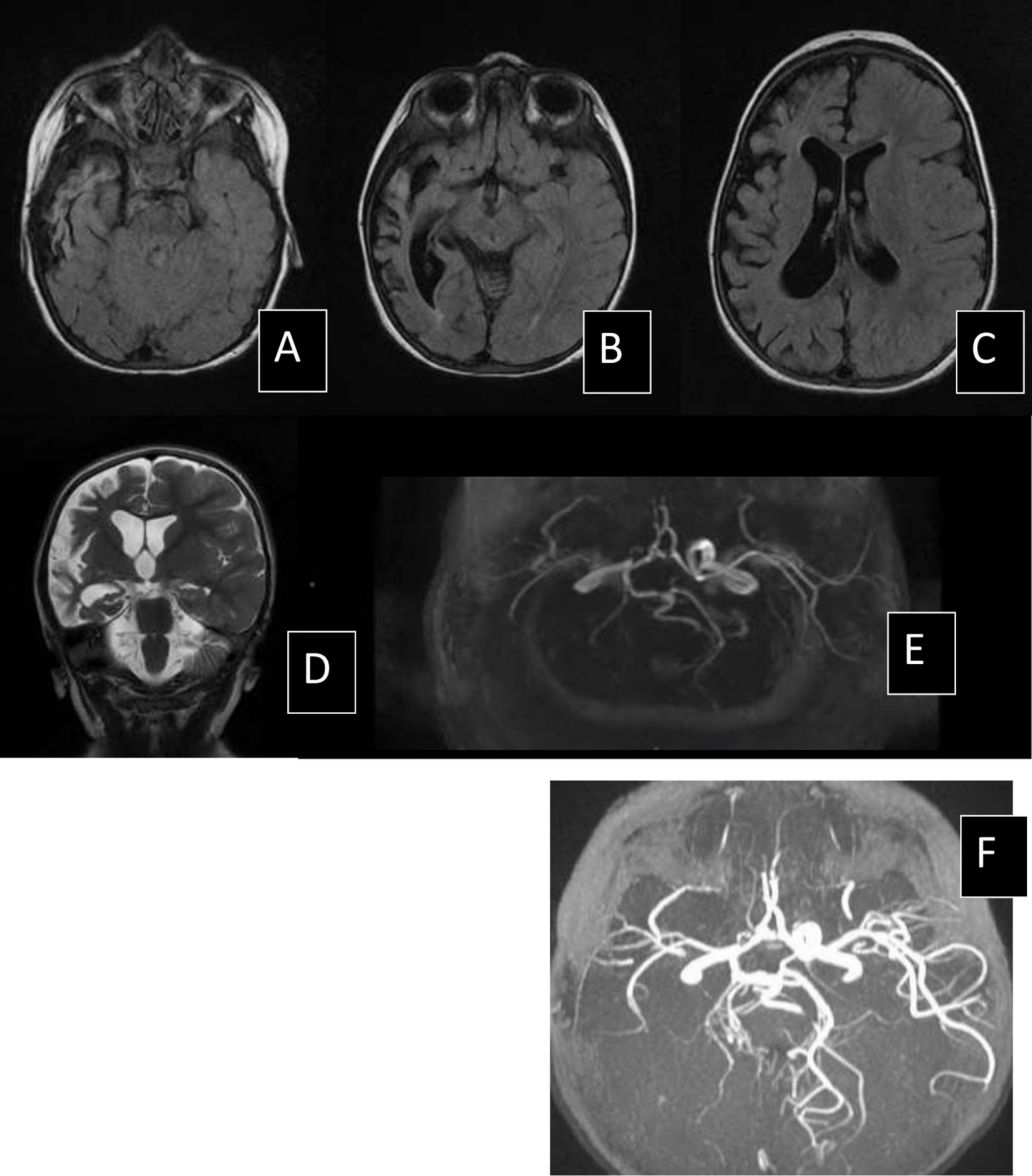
MRI scan of patient N09, 1 year after stroke secondary to cerebral VZV vasculitis. Right hemisphere atrophy on axial FLAIR sequence (A–C) and coronal T2 sequence (D) and stenosis of internal carotid artery and posterior cerebral arteries in vascular sequences Time of flight (TOF) angiography (E, F).
